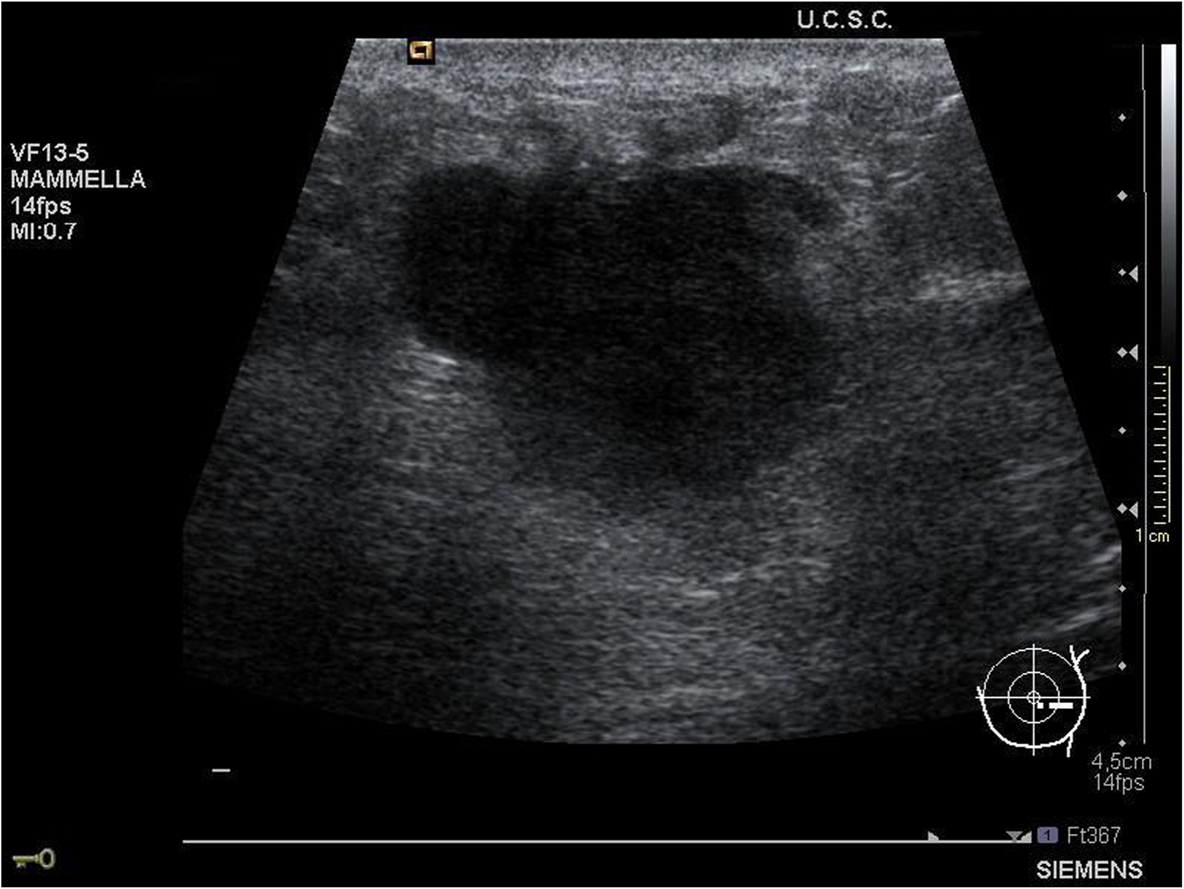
Radiological aspect. Breast ultrasonography showed a solid non-homogeneous hypoechoic lesion with partially regular contour
